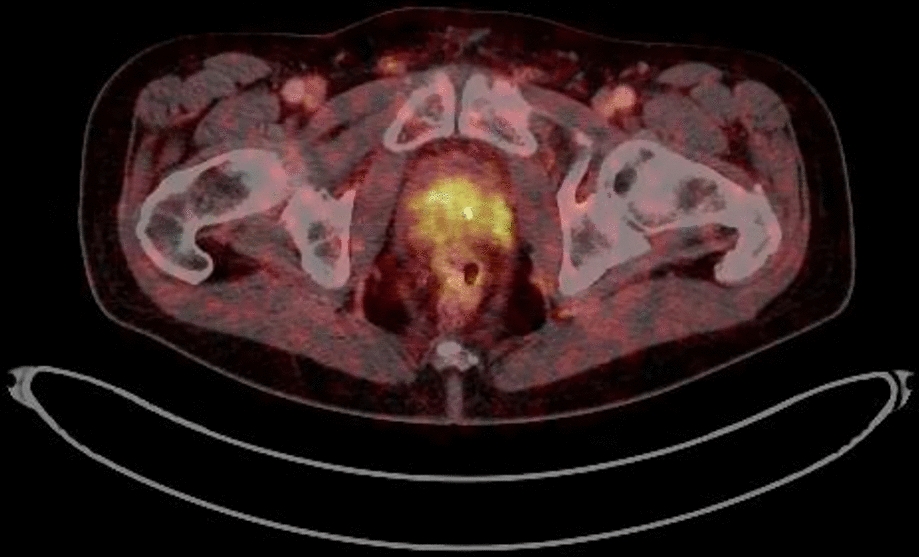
PSMA-tracer uptake of the prostate gland
